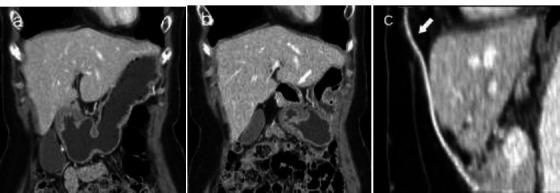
Abdomen CT in a patient who underwent LHPOF.
